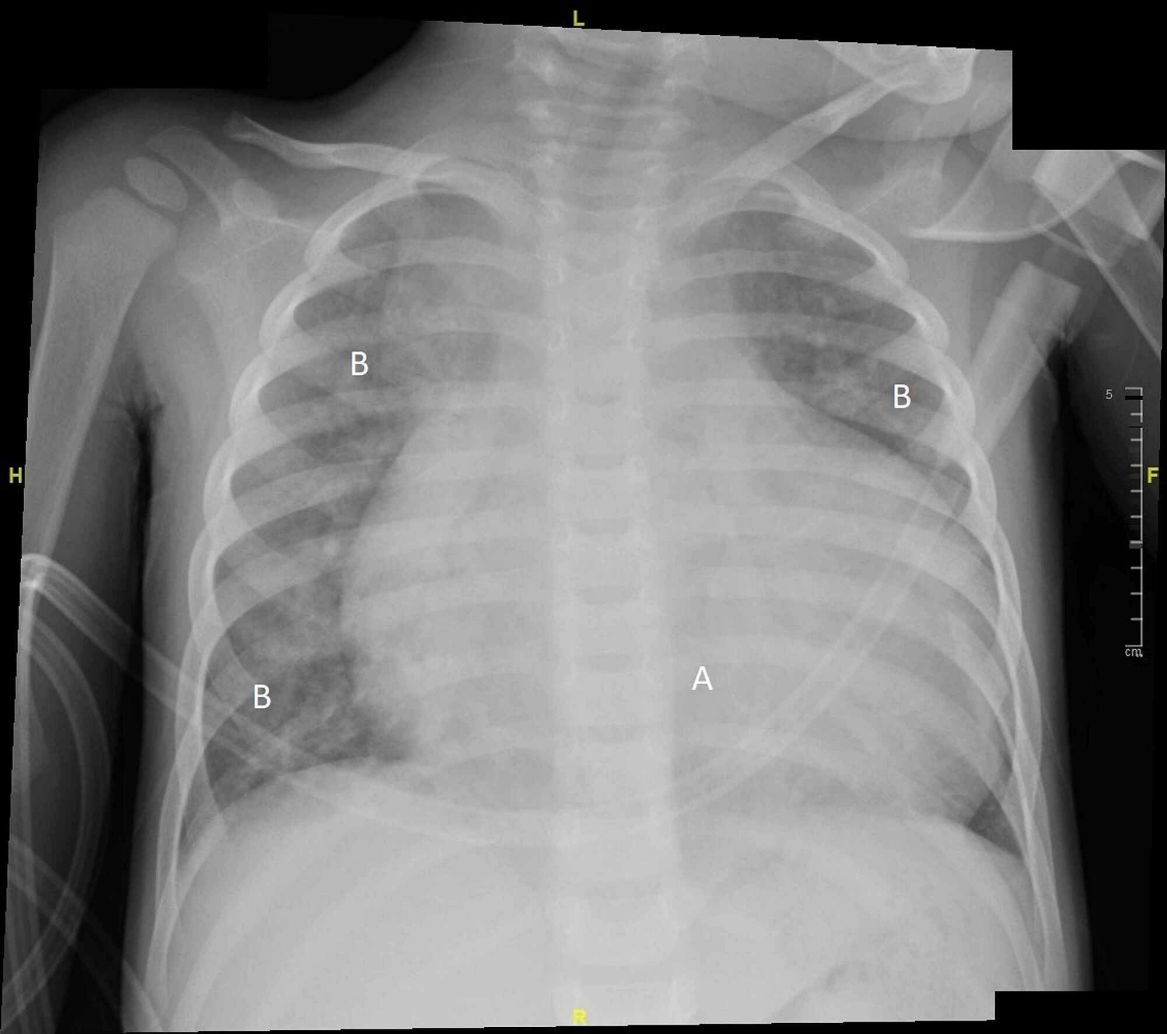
Initial CXR in the ED showing consolidation and cardiomegaly. A: cardiomegaly; B: lung consolidation CXR, chest X-ray
